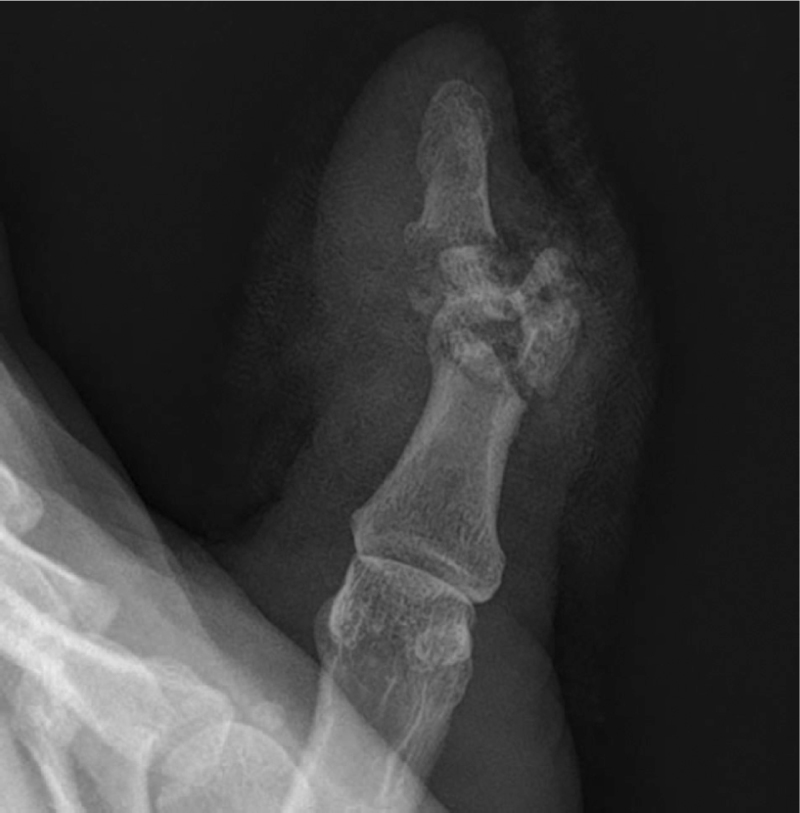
Preoperative oblique x-ray of the affected finger.
